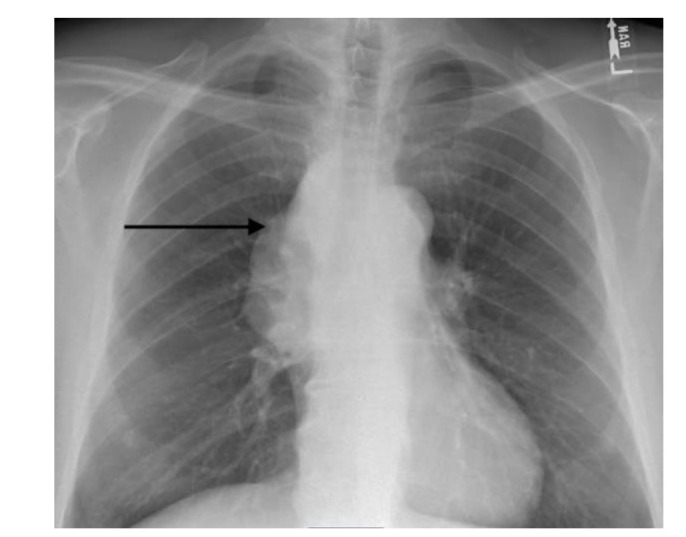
Chest radiograph suspicious for right mediastinal mass
